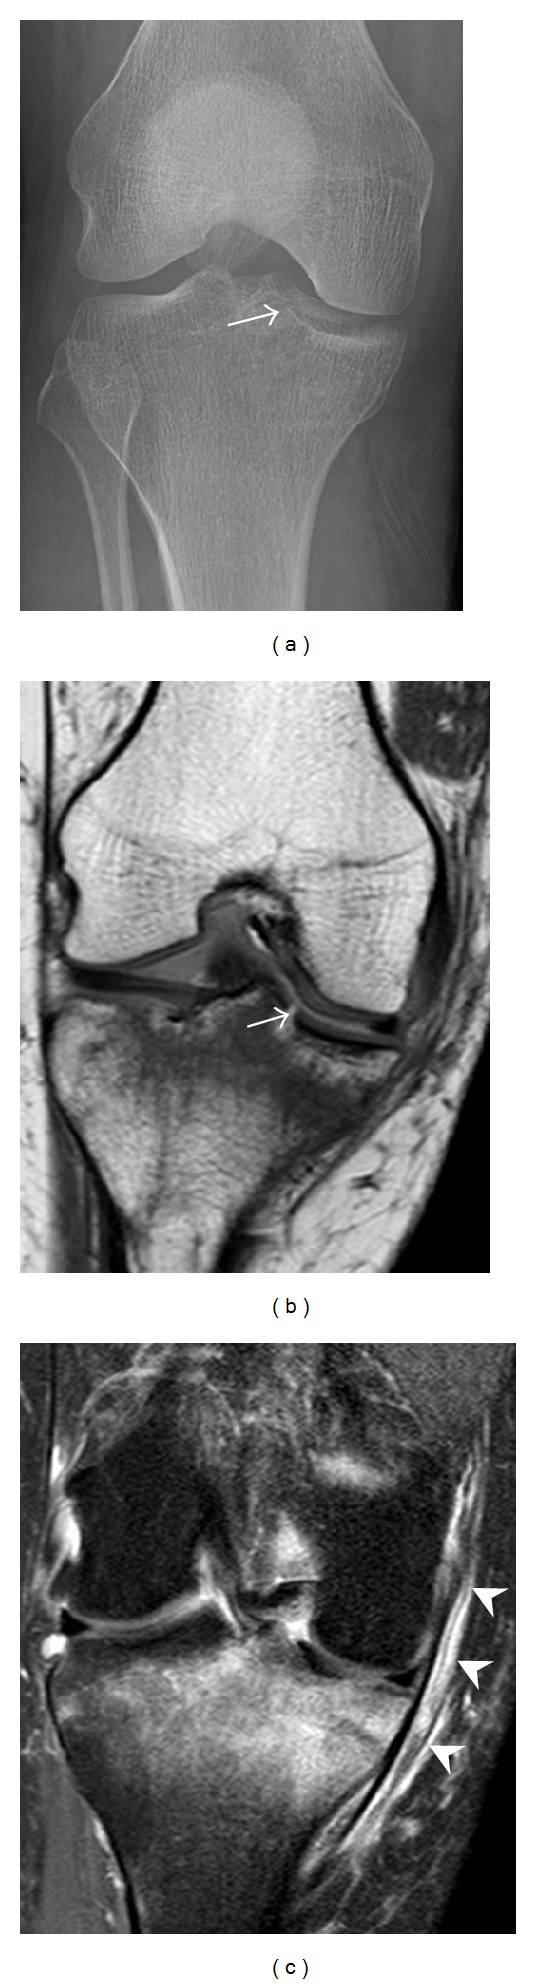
A 56-year-old woman presenting with left knee pain after a fall. (a) Initial anteroposterior radiograph was considered normal, however, subtle cortical disruption of the anterior rim of the medial tibial plateau, medial to the tibial spine, is noted (arrow). (b) Coronal T1-weighted MRI confirms the cortical disruption (arrow) and shows extensive fracture through the proximal tibia. (c) Coronal proton density-weighted image with fat saturation shows extensive edema in the subchondral bone. Note also hypersignal adjacent to the medial collateral ligament corresponding to a grade I sprain (arrowheads).
